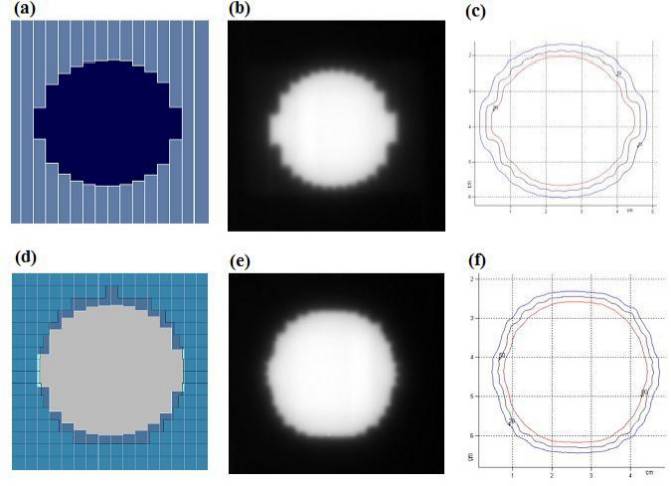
Circular field shaping by single‐layer and dual‐layer leaves of dual‐layer micro‐multileaf collimator (DmMLC). (a) Beam‐eye view (BEV) using single‐layer leaves of the DmMLC. (b) Radiograph by single‐layer leaves of DmMLC. (c) Isodose lines of circular field shaped by single‐layer leaves of DmMLC. (d) BEV of dual‐layer leaves of DmMLC. (e) Radiograph by dual‐layer leaves of DmMLC. (f) Isodose lines of circular field shaped by dual‐layer leaves of DmMLC.
